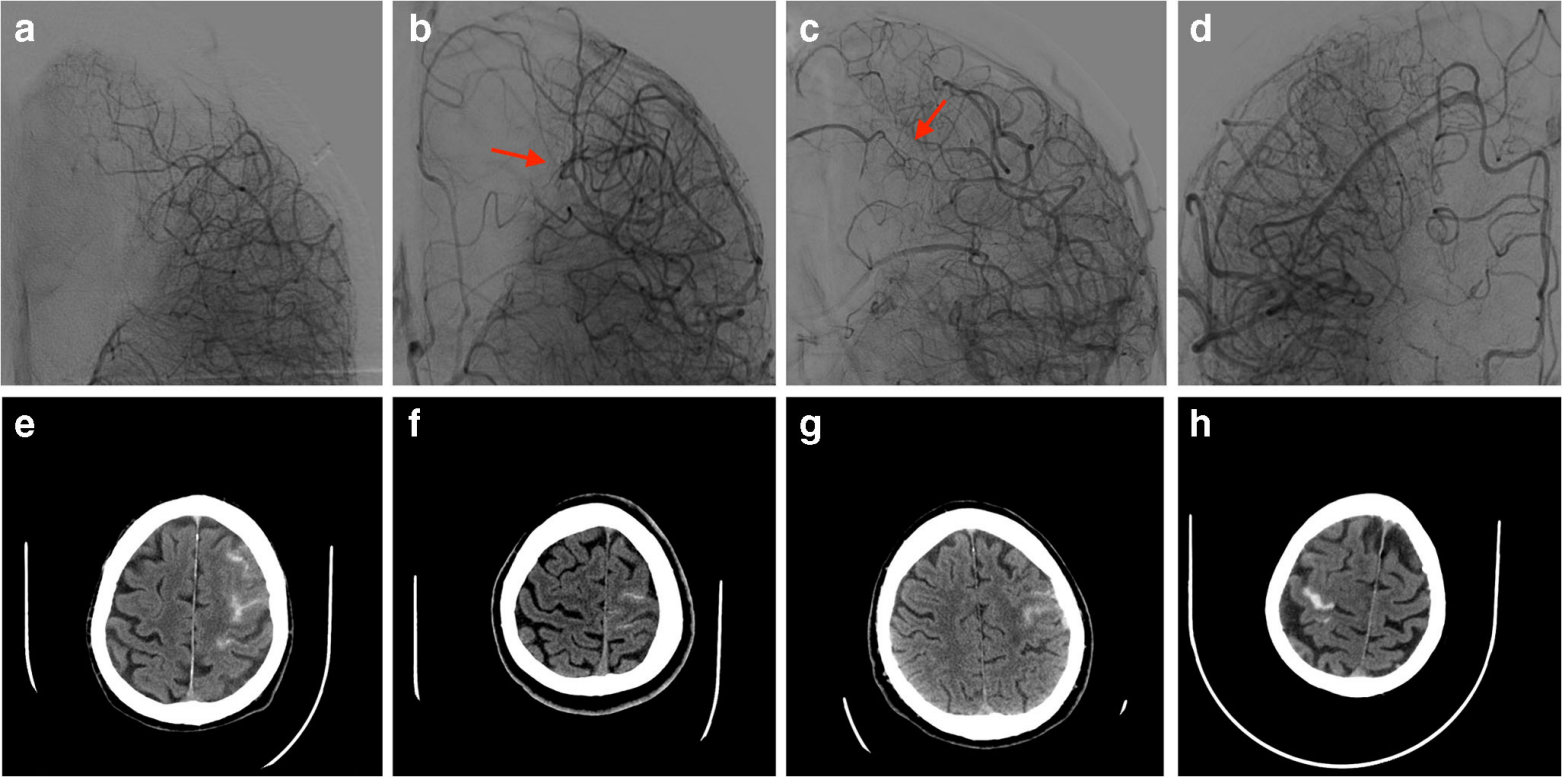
Cases 1/2/3/4. a–d Capillary phase of the angiographic control after stenting showing the hemodynamic overload of leptomeningeal arteries and the inversion of flow in two patients (red arrow). e–h CT control showing watershed subarachnoid hemorrhage
